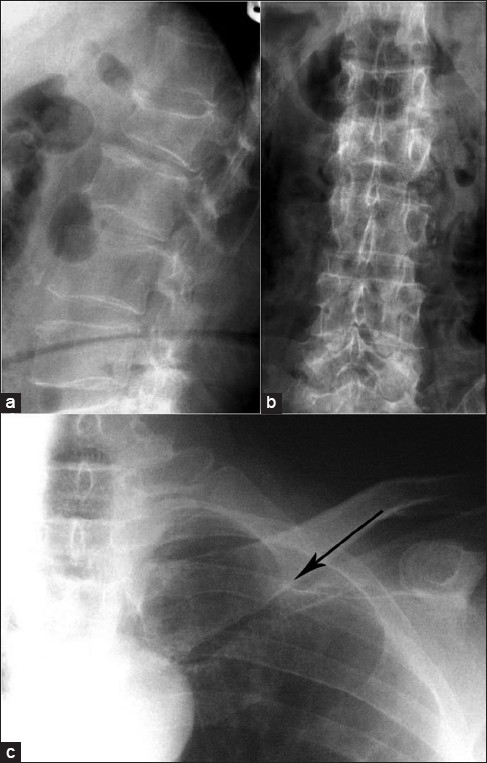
X-ray lumbosacral spine lateral view (a) and anteroposterior view (b) showing compression fracture of L2 vertebrae. X-ray of (L) shoulder with clavicle shows a thin hair line fracture at the medial end of left clavicle (solid arrow)
